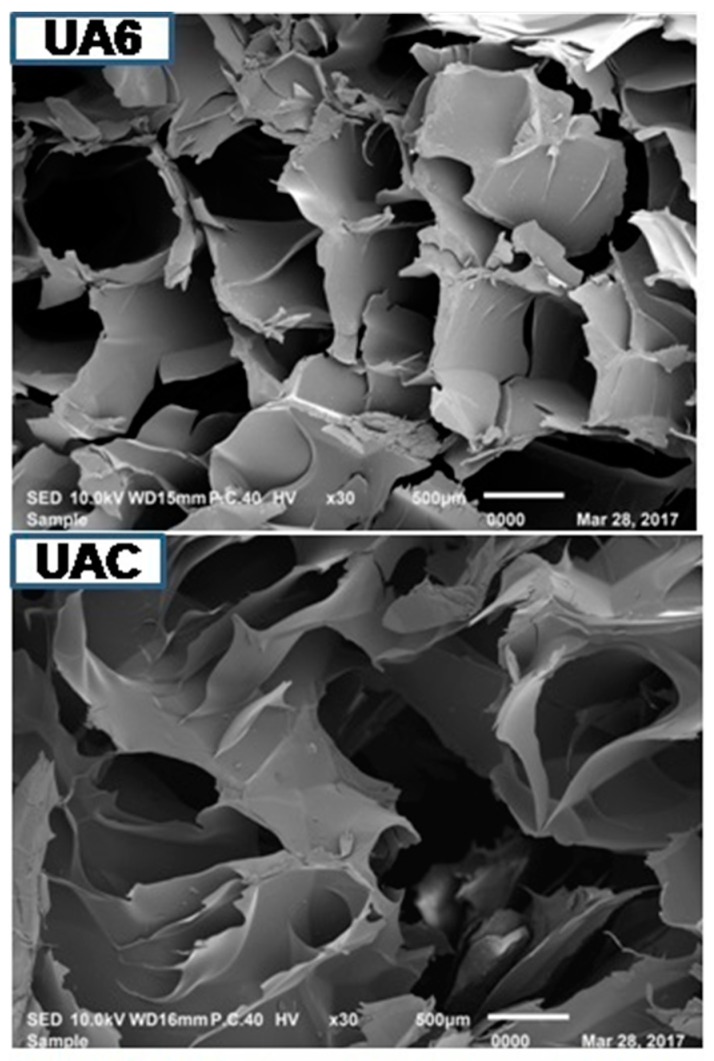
SEM images of gelatin extracted using ultrasound for the time duration of 2, 4 and 6 h (UA2, UA4 and UA6, respectively) with actinidin pretreatment. UAC: control gelatin extracted using ultrasound without enzyme pretreatment.
